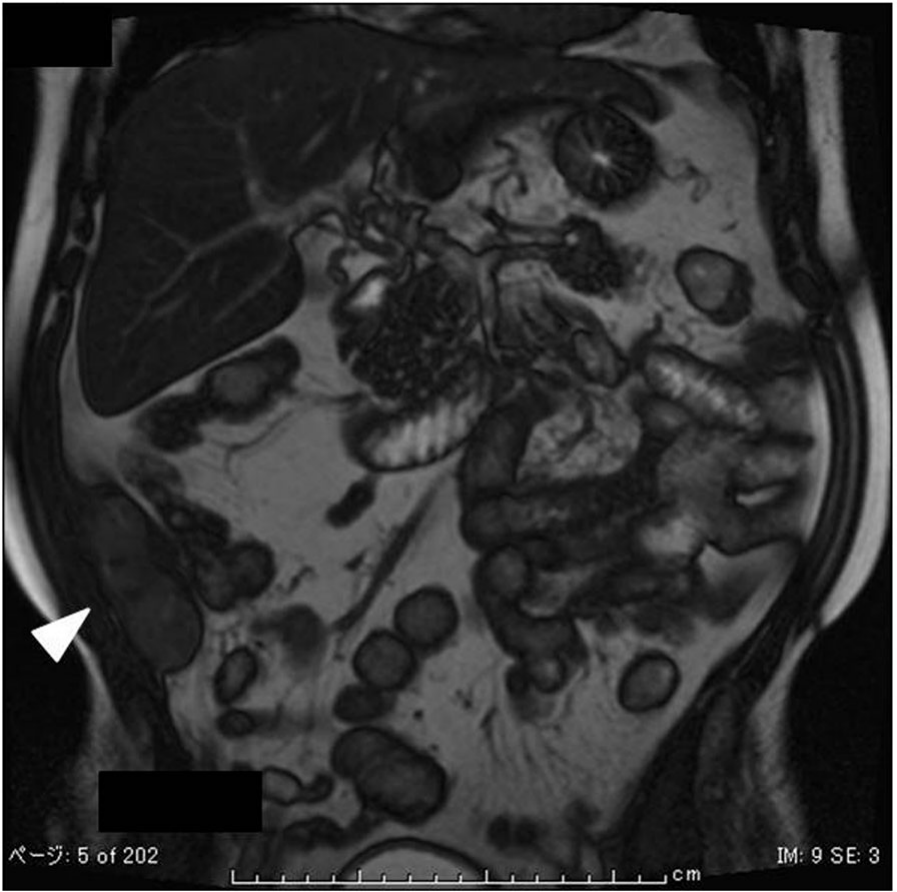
Magnetic resonance imaging of the abdomen showing elliptical masses in the transverse abdominal wall exhibiting low intensity on T2-weighted images (arrowhead)
